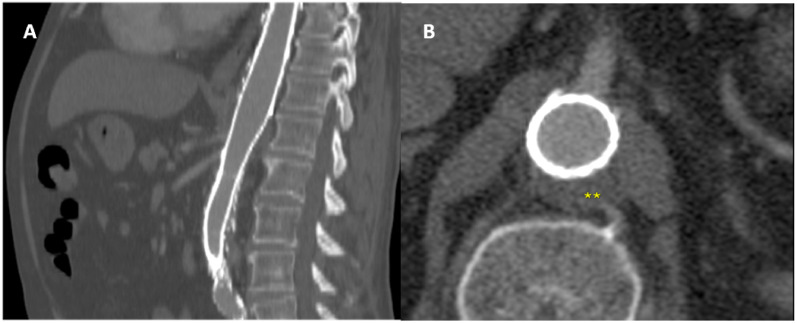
Fig. 3 Sagittal (A) and axial (B) computed tomography images showing the Cardiatis multilayer correctly deployed and the complete thromboses of the posterior visceral penetrating aortic ulcer.
